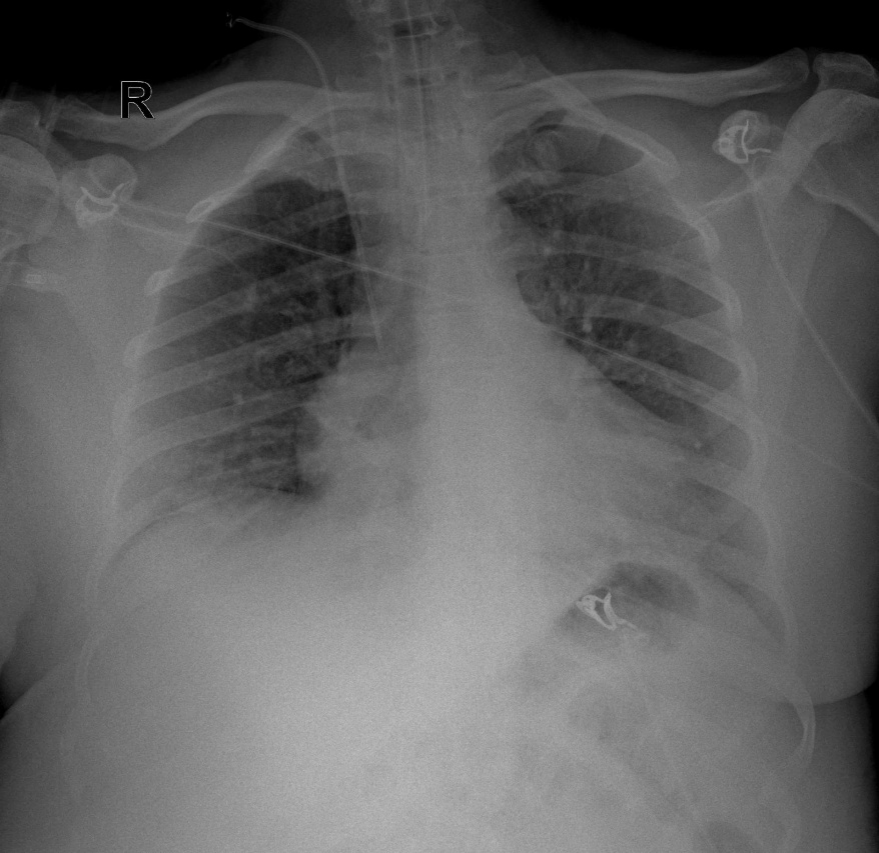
Anteroposterior chest X-ray performed on day 12 of ICU stay (day 12 of ARDS evolution) and day 4 after extubation, showing significant improvement in lung aeration.
